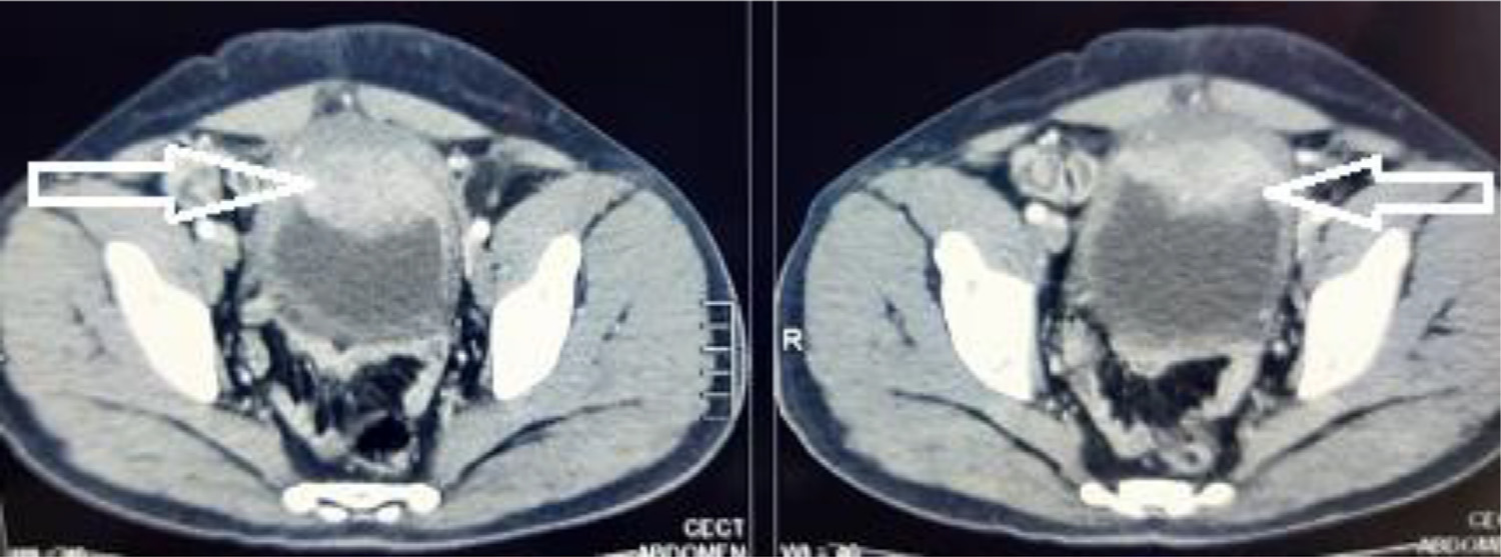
Axial contrast CT showing an irregular 7 × 8 cm enhancing mass related to dome of bladder (white arrow) with surrounding fat stranding.
